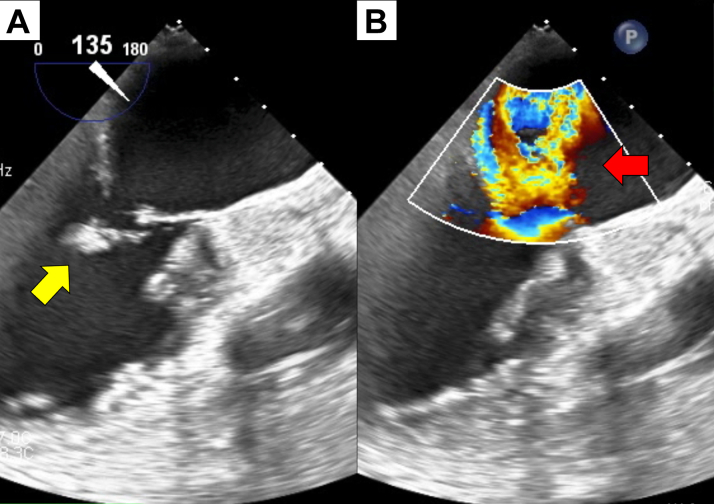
Severe Mitral Regurgitation Due to Papillary Muscle Rupture (A) Transesophageal echocardiography demonstrating the presence of papillary muscle rupture (yellow arrow). (B) Transesophageal echocardiography demonstrating the presence of severe mitral regurgitation (red arrow).
